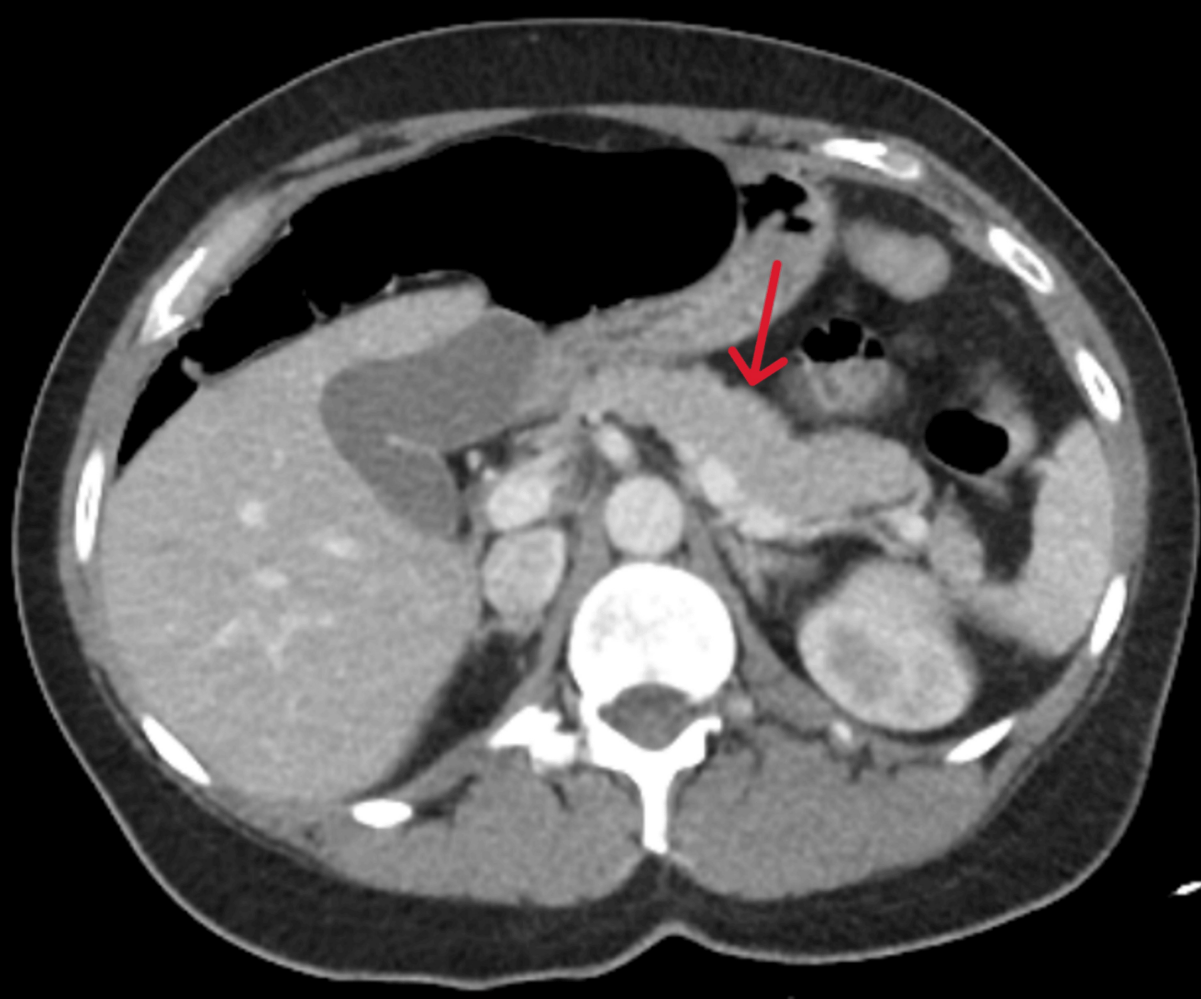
Axial abdominal CT CT scan of the abdomen and pelvis shows mild diffuse pancreatic parenchymal swelling with minimal changes in density suggestive of mild pancreatitis. No evidence of necrosis, pseudocysts, or abscesses in the pancreas or surrounding tissues. Otherwise unremarkable CT of the abdomen and pelvis. CT: computed tomography
